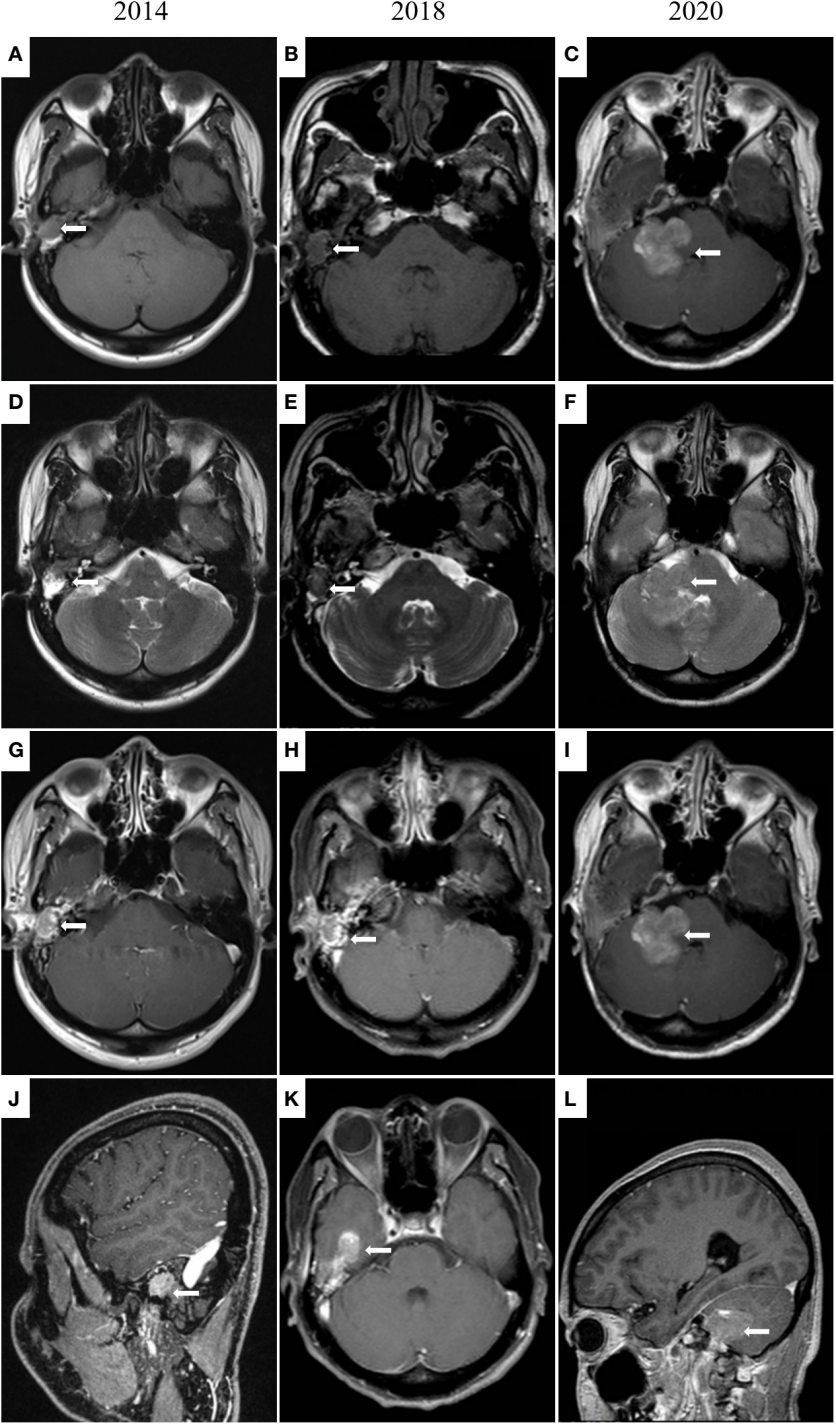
MRI images of the middle ear and brain. In 2014 (A, D, G, J), irregular soft tissue signal shadows in the right external auditory canal, middle ear cavity, and mastoid cells are shown. In 2018 (B, E, H, K), an irregular mass (14 × 18 × 12 mm) in the right external auditory canal and middle ear cavity and tumor invasion into the brain is presented. In 2020 (C, F, I, L), tumor metastases in the right cerebellopontine angle and right temporal meningeal.
